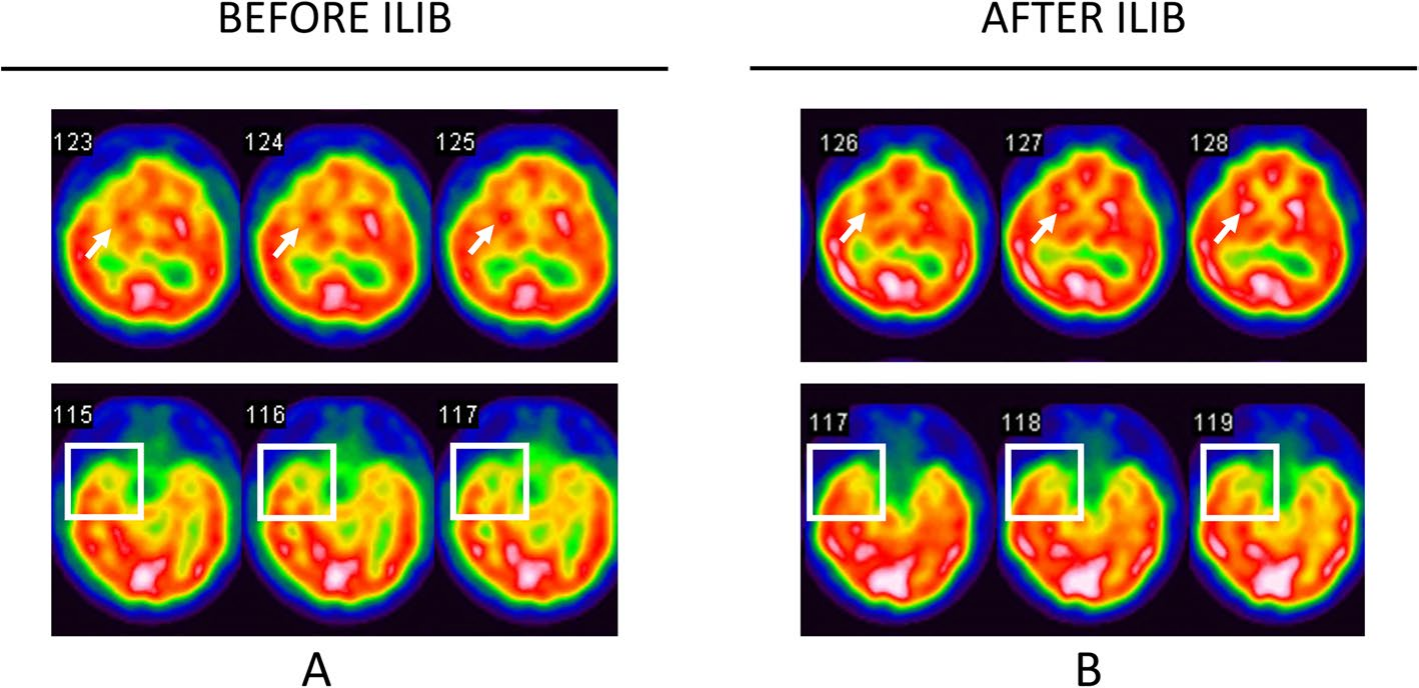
The brain SPECT images of the patient. In A, SPECT showed a decrease of the right cerebral blood flow in the right sensorimotor strip, frontal lobe, anterior and posterior cingulate gyri, left medial temporal lobe (white box), corona radiata, basal ganglion (white arrow), and thalamus. In B, there is increased uptake in the frontal lobe, temporal lobe, basal ganglion, amygdala, and pons
